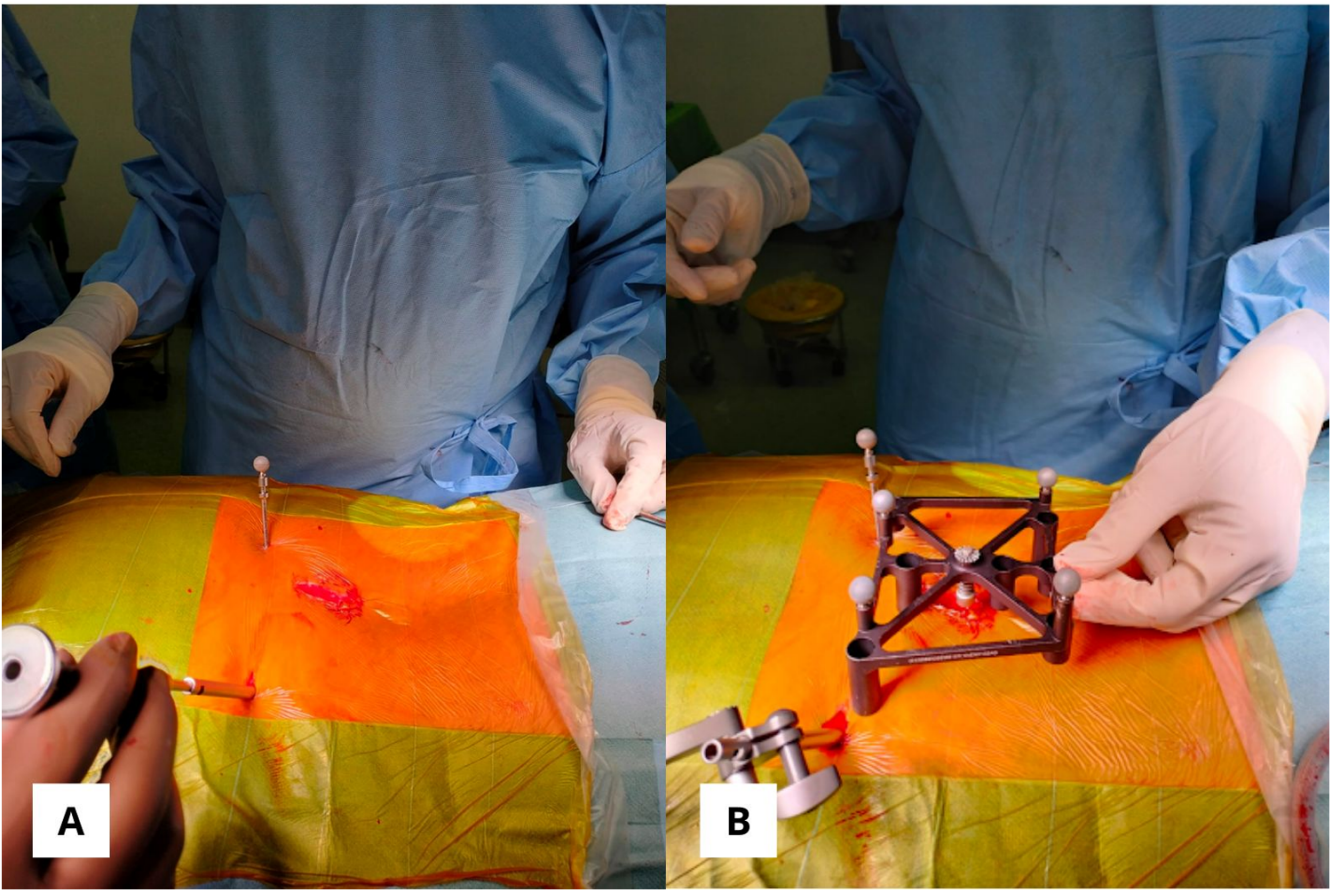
(A) The posterior superior iliac spine bilaterally is used for dynamic reference base and surveillance marker positioning. (B) ICT (Intraoperative Cone-Beam Tomography) was attached to the spinous process without touching the skin.
